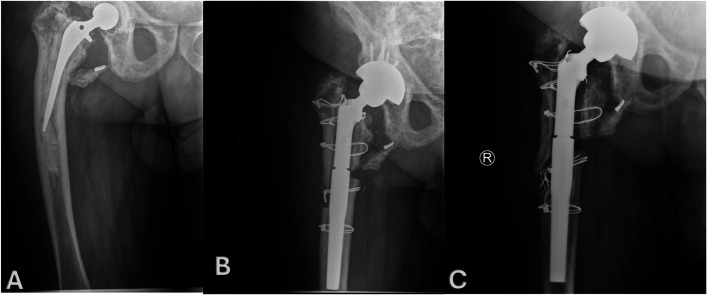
A 74-year-old man with underlying Paget disease who underwent ETO combined with medial corticotomy for revision THA due to femoral varus deformity. Fig. 4-A Preoperative AP radiograph demonstrating the varus femoral deformity with fracture and displaced right lesser trochanter containing a broken screw. Fig. 4-B One-month postoperative AP radiograph showing the ETO and medial corticotomy with correction of femoral varus deformity. Note the persistent gaps between the trochanteric fragment and the femoral shaft. Fig. 4-C Twelve-year postoperative AP radiograph showing the nonunion of medial corticotomy, ETO, and trochanteric fragments. AP = anteroposterior, ETO = extended trochanteric osteotomy, and THA = total hip arthroplasty.
